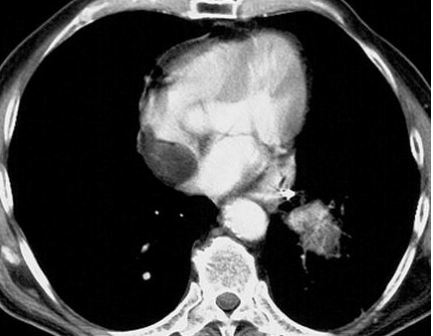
Select axial image of computed tomography showing a right atrial lipoma.
